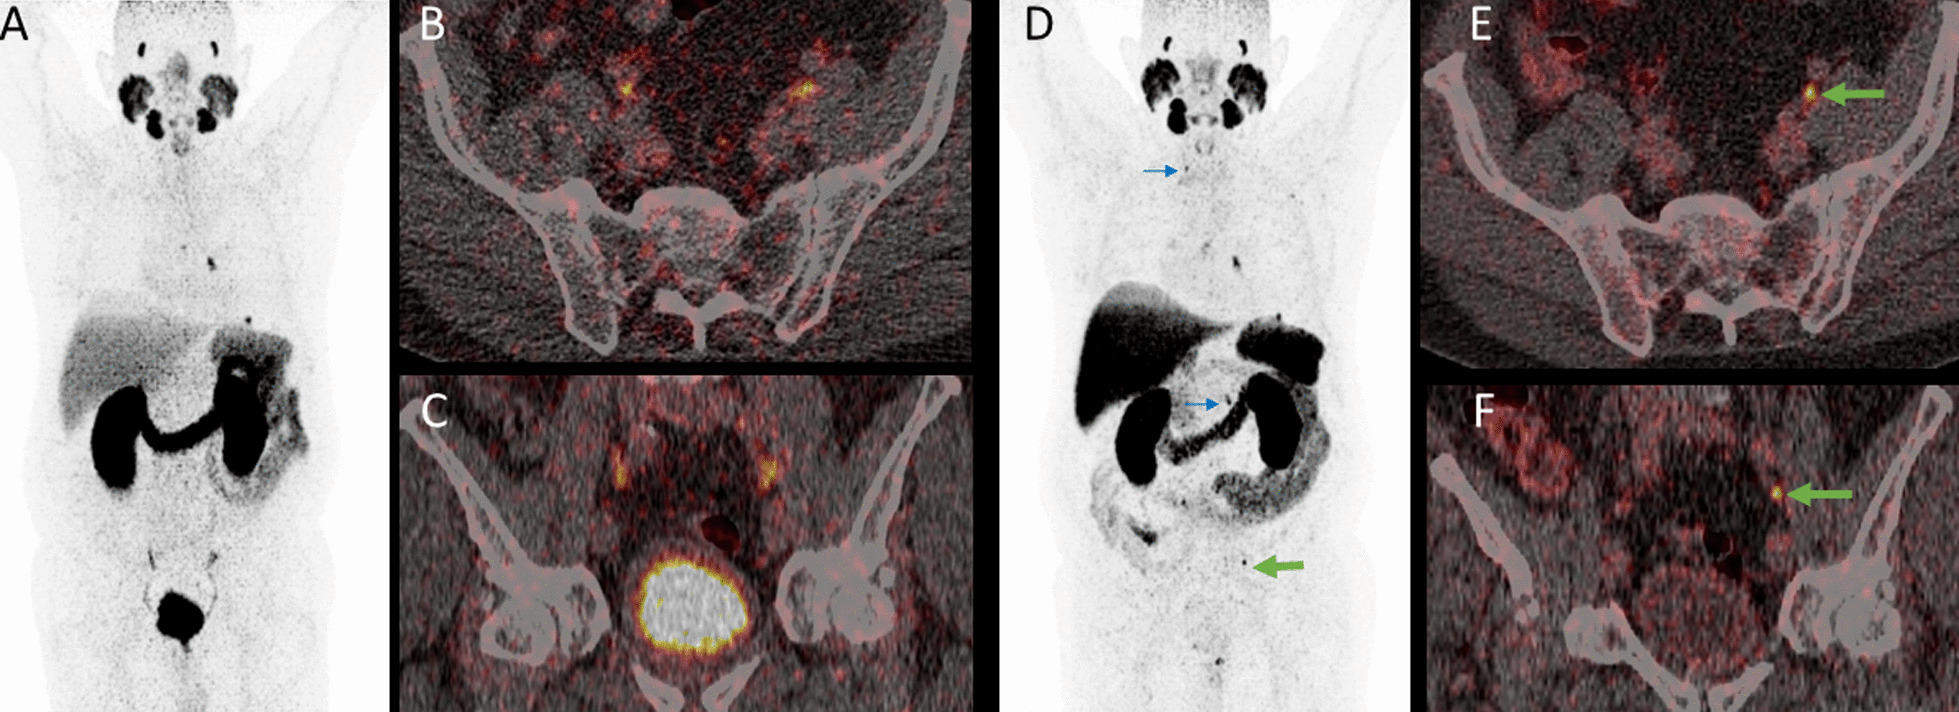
MIP of [^68^Ga]-Ga-PSMA-11 PET (A) with quite similar longitudinal uptake in both ureters. In both axial (B) and coronal fused (C) PET/CT images, an accessory paraureteral lesion is not detectable. In [^18^F]-F-PSMA-1007 PET, the paraureteral iliac extern lymph node metastasis is clearly depictable in both the MIP (D) and the axial (E) and coronal fused (F) PET/CT images (green arrow). Please note several other concordant malignancy-associated findings: local tumor recurrence in the left prostatic lobe, infracarinal and left-hilar lymph node metastases. Additionally, there is a small focus in the left lower lobe of the lung (in detail in Fig. 7). Auxiliary findings: presumable unspecific uptake in cervical and coeliac ganglia (blue arrow) in [^18^F]-F-PSMA-1007 PET/CT. Unspecific ileocoecal uptake is also present in [^18^F]-F-PSMA-1007 PET
